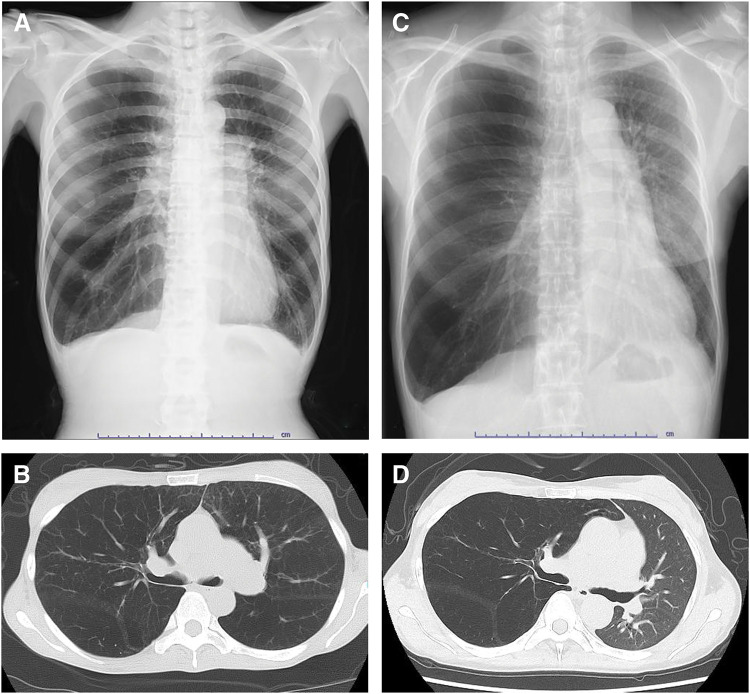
(A) Preoperative chest X-ray. (B) Preoperative chest computed tomography. (C) Chest X-ray during the first 6 months after lung transplantation. (D) Computed tomography during the first 6 months after lung transplantation.
